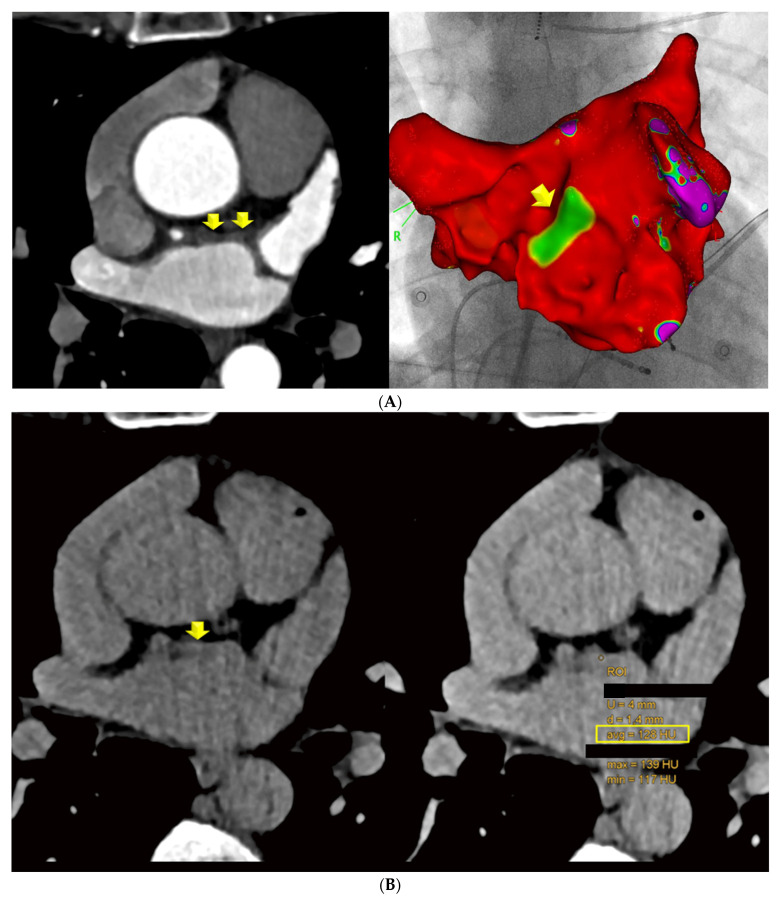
A 59-year-old-male with paroxysmal AF, a BMI of 21.5, the CVRF of dyslipidemia, and left atrial wall thickness (LAWT) of 3.3 mm, with a low-voltage area on EAM (anterior LA wall, green/yellow area) (upper panel, (A)) matching with focal LE (128 HU) on late enhancement (LE) CT (lower panel, (B)).
